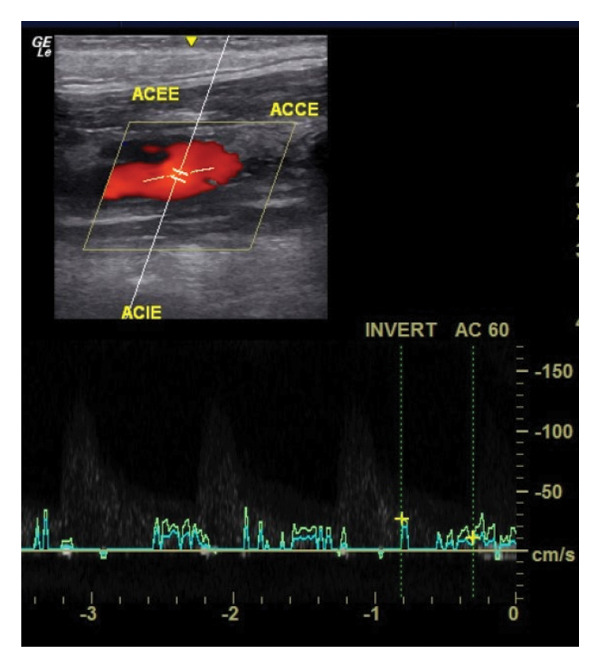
Postoperative Doppler performed at 30 days. (ACCE: left common carotid artery. ACIE: left internal carotid artery. ACEE: left external carotid artery).
